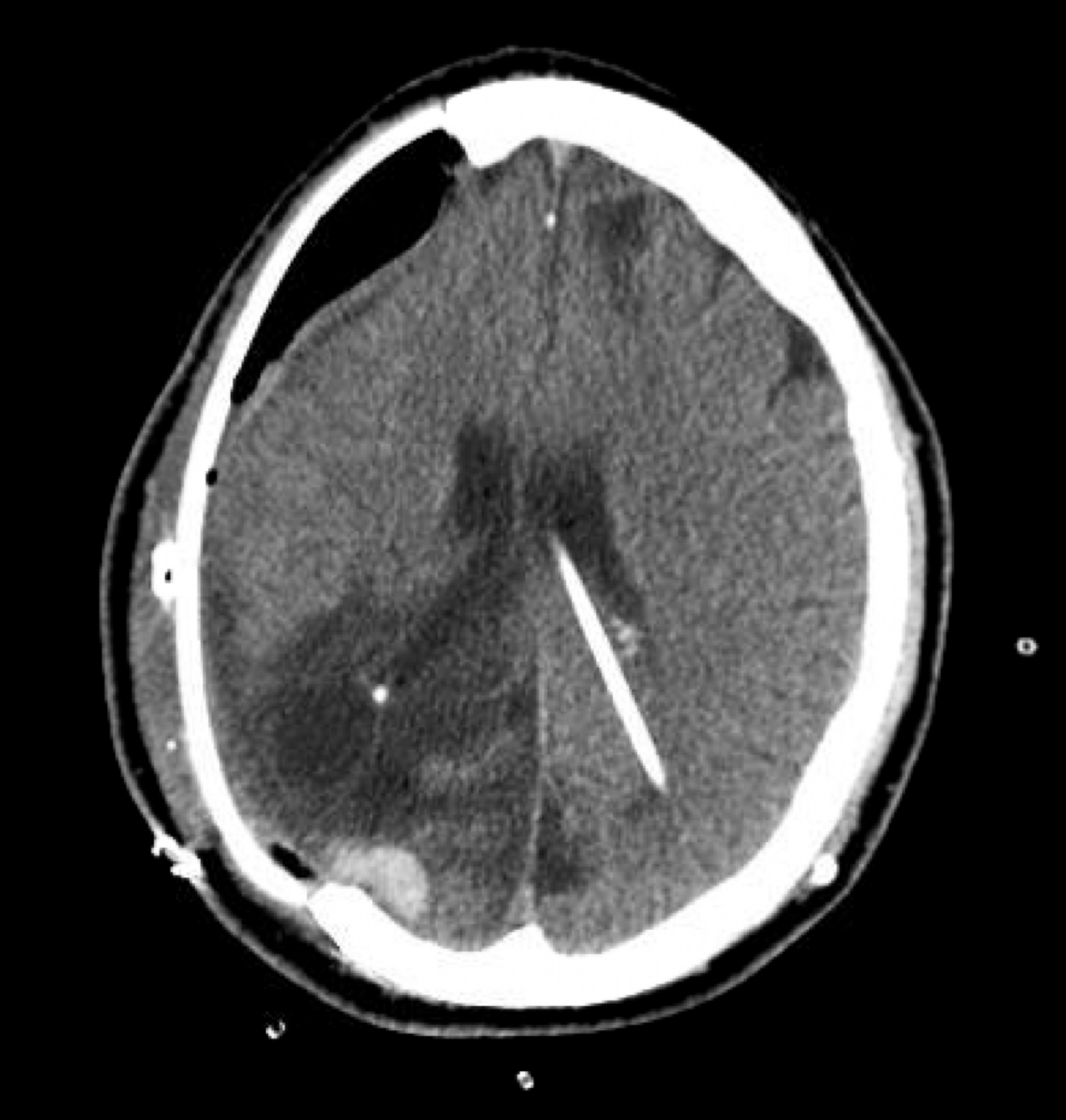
Primary cranioplasty surgery post-op CT imaging CT, computed tomography
